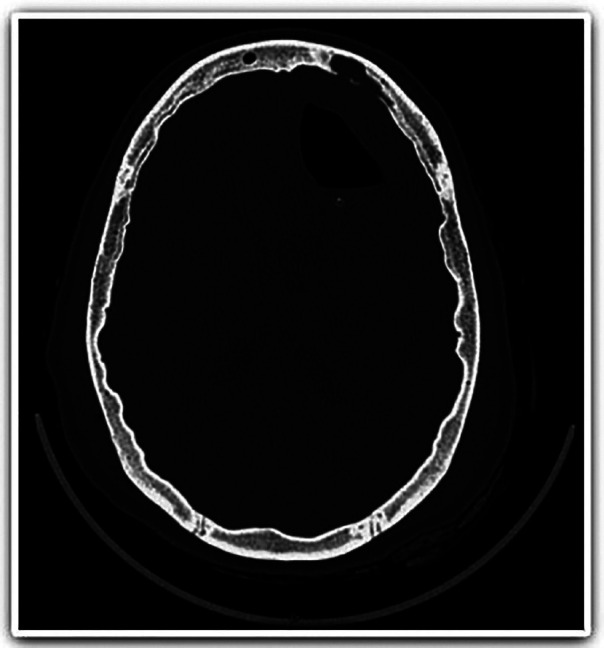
MRI revealing hyposignal compatible with brain abscess.
